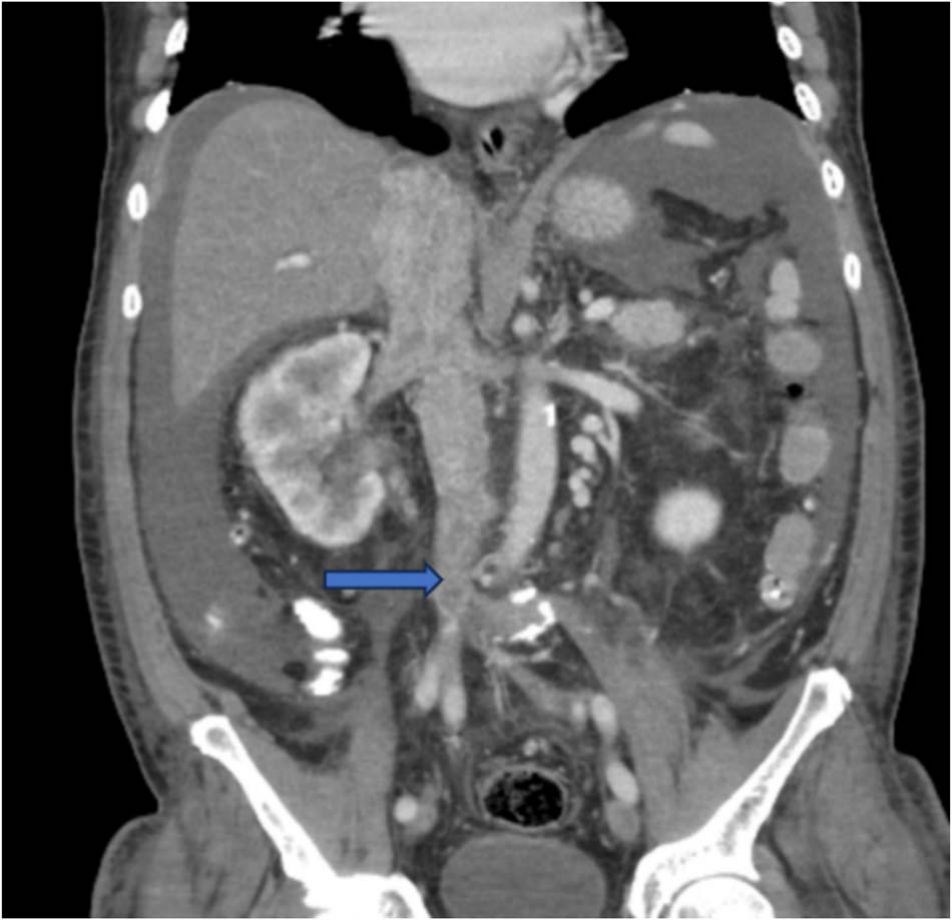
CT chest abdomen and pelvis with contrast showing inferior extension to the level of the distal margin of the IVC.
